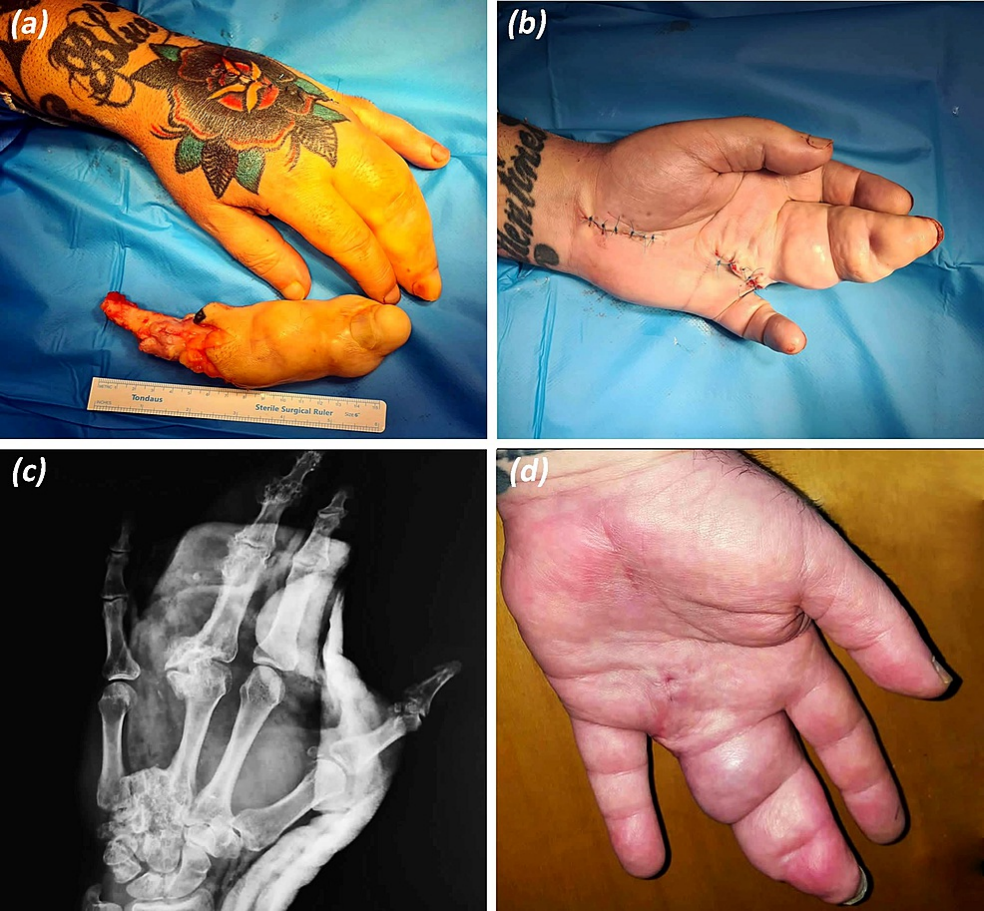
Postoperative images and at follow-up. (a) Ray resection at the proximal base of the fourth metacarpal. (b) Racket-shaped incision for the resection of the fourth ray and palmar incision for carpal tunnel release. (c) Postoperative X-ray with hand in splint. (d) Palmar view six months postoperatively, the patient reported good aesthetic and functional results.
